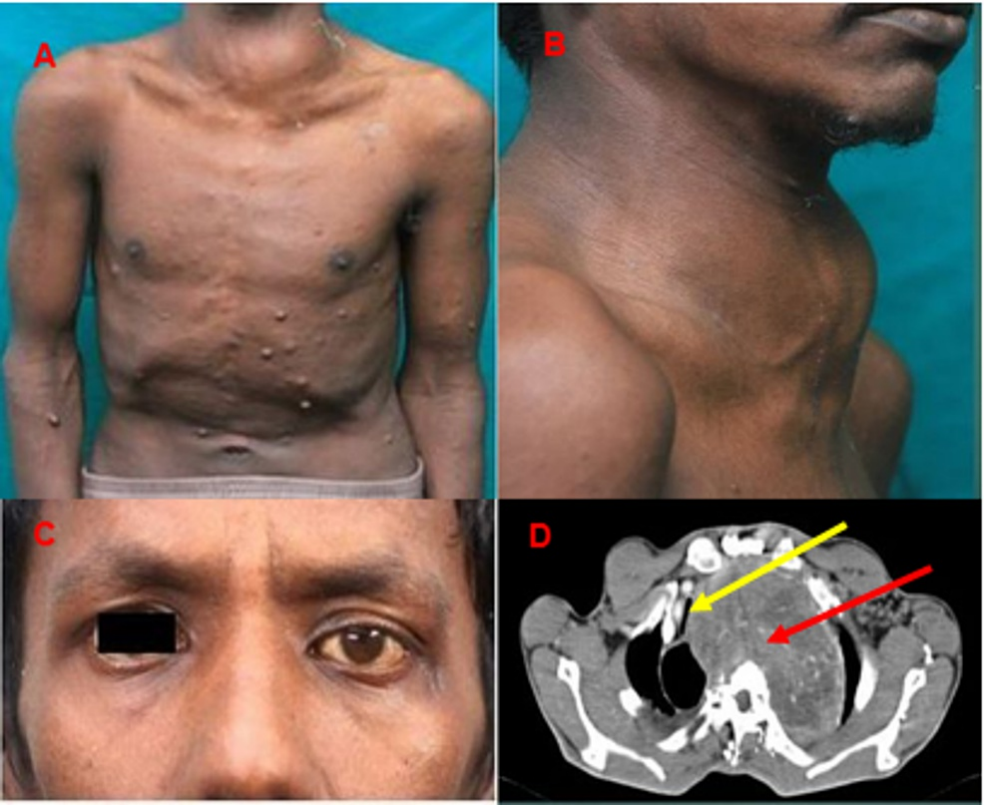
Clinical and radiological findings. The clinical photograph (A) shows multiple nodules over the chest wall, arms, and forearms consistent with neurofibromatosis. (B) shows a large swelling over the anterolateral aspect of the neck on the left side. (C) shows meiosis and partial ptosis of the left eye. CT scan shows a large lobulated mass (red arrow) compressing the trachea (yellow arrow) giving a slit-like appearance (D).
